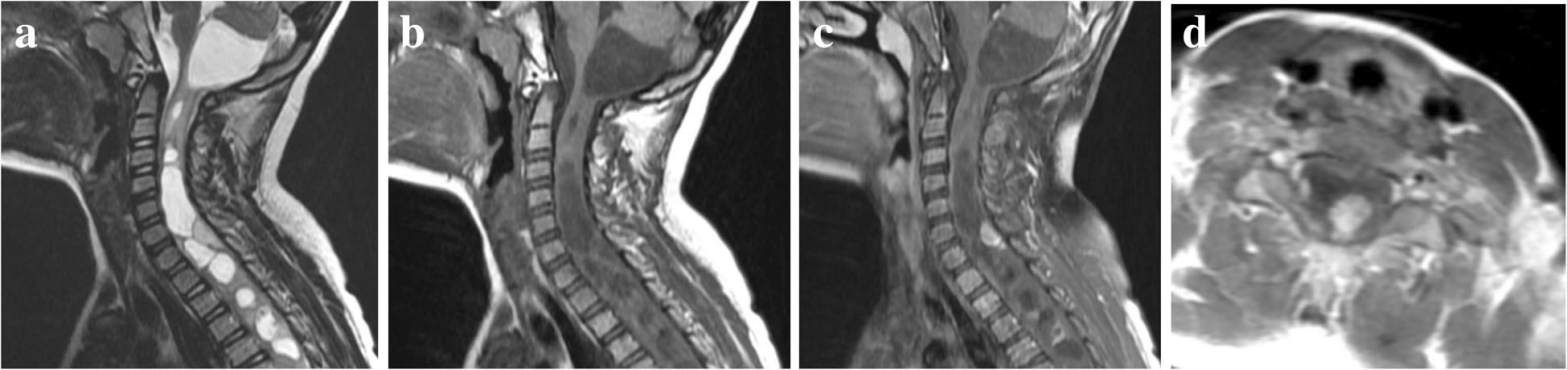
Cervical-thoracic PA. a, Preoperative sagittal T2-weighted MR image shows a solid-cystic lesion with multi-segmental involved of spinal cord. b, Sagittal T1-weighted MR image shows the solid component of lesion at hypointense signal intensity. c, Sagittal contrast-enhanced T1-weighted MR image shows focal nodular enhancement of solid component. d, Axial contrast-enhanced T1-weighted MR image shows that the solid nodule is obviously enhanced. The cystic wall demonstrates no enhancement
